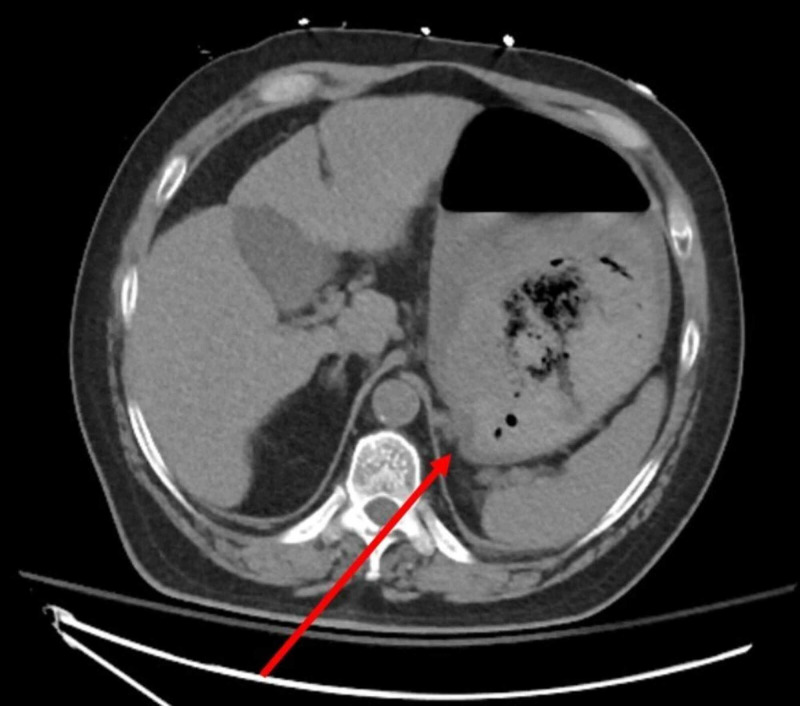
CT scan of the abdomen The red arrow shows gastric varices.
